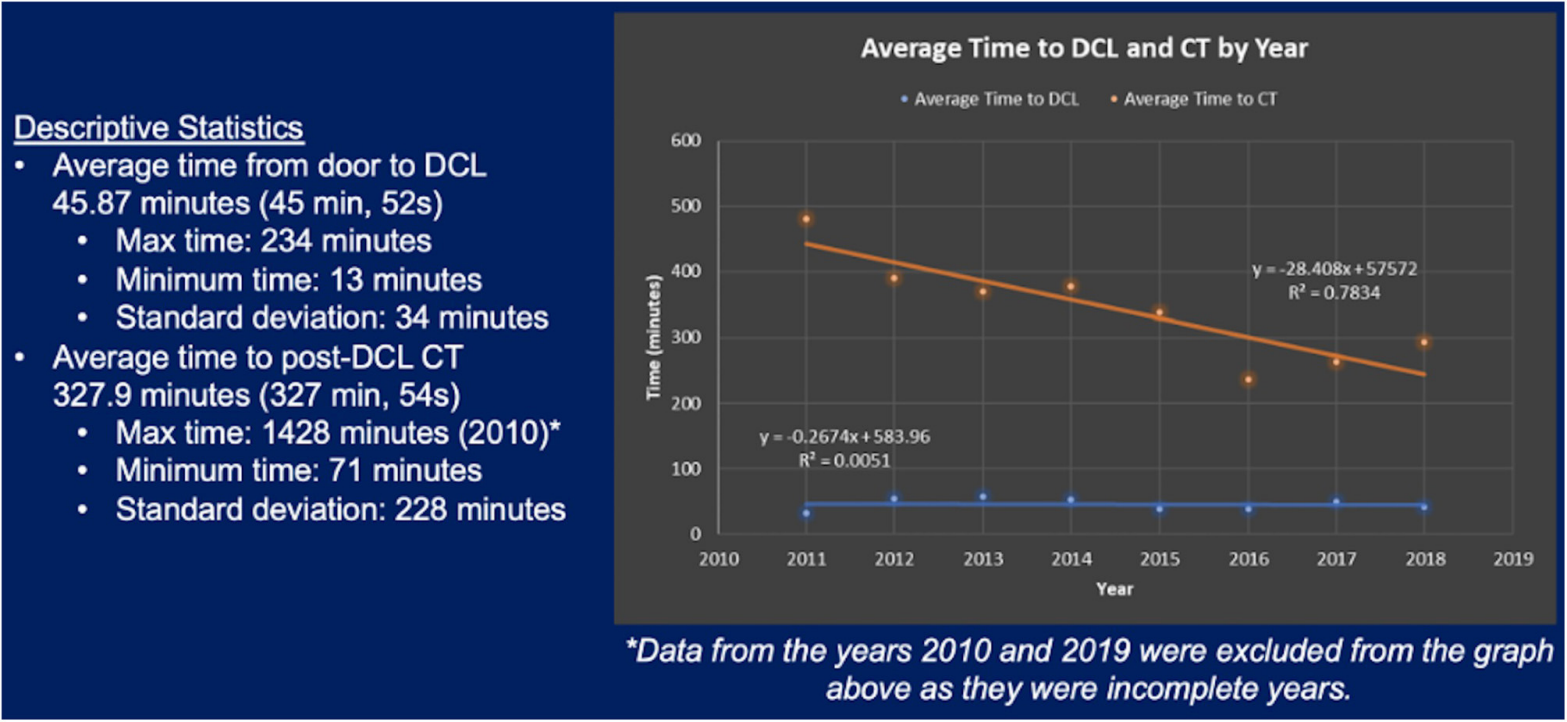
Average time from door to DCL and average time to post-DCL CT between January 2011 and December 2018.
